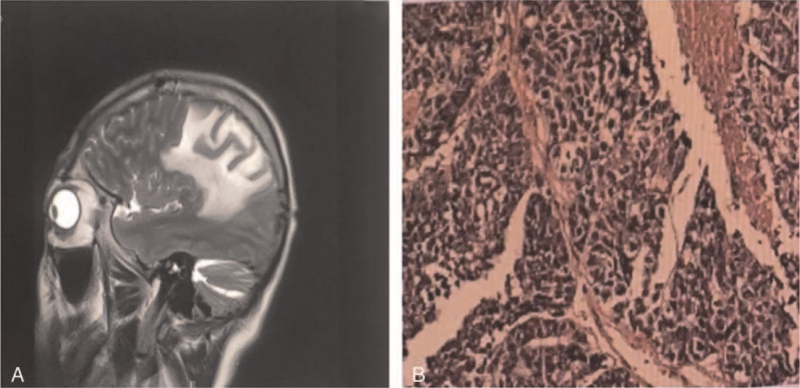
A) Irregular mixed signal clumps (approximately 4.3 × 2.0 cm) can be seen on the left parietal lobe. T1-weighted, T2-FLAIR and DW1 show high and low mixed signal shadows. The left ventricle was compressed, and the midline structure was shifted to the right. B) The space-occupying lesions were metastatic poorly differentiated squamous cell carcinoma. Immuno Histo Chemistry (IHC) 67(+70%), CKpan (+), CD56(−), Syn(−), CgA(−), TTF-1(−), CK5/6(+), LCA(−), P63(+), GFAP(−), Oligo-2(−).
